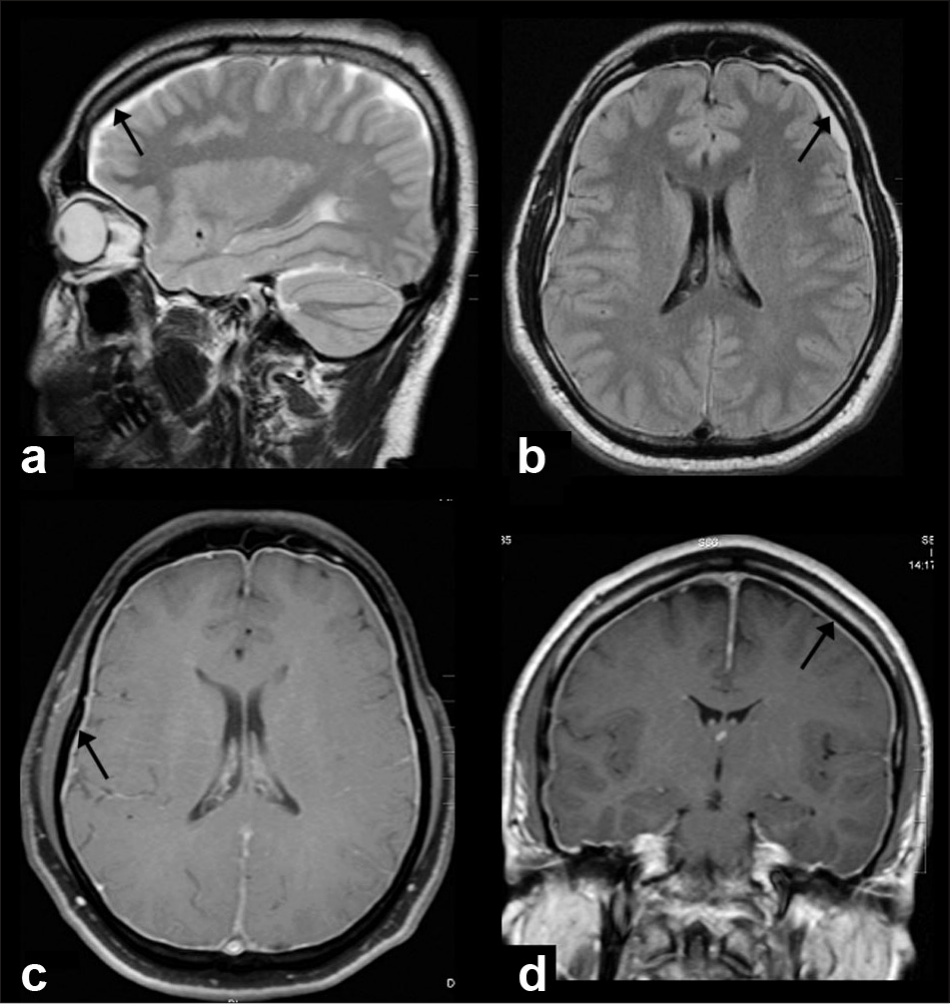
Sagittal T2 weighted (a) and axial FLAIR (b) images show uniform hyperintensity of pachymeninges (arrows). Axial fat suppressed postcontrast (c) and coronal postcontrast (d) images show diffuse pachymeningeal gadolinium enhancement
